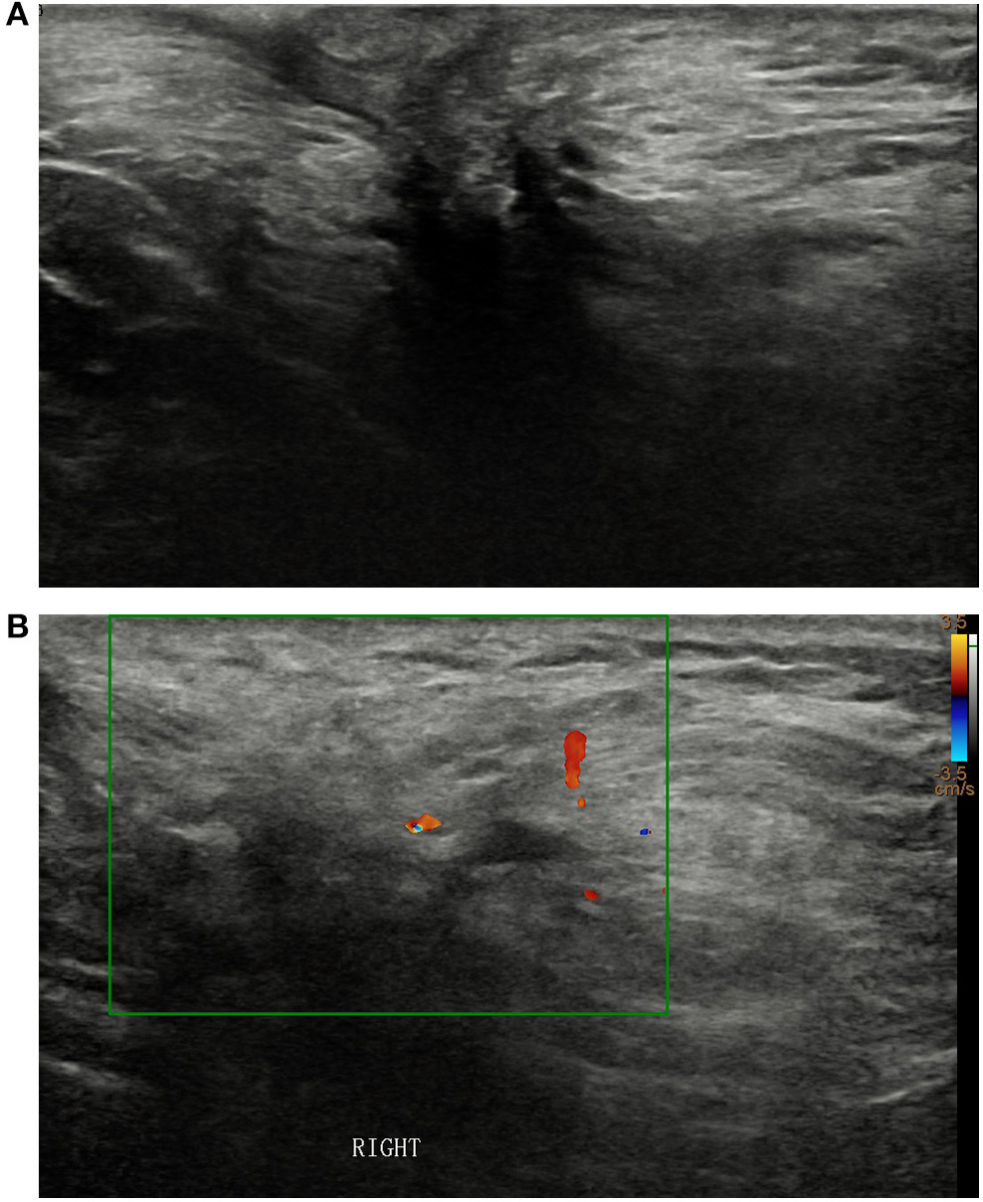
(A) Sonographic examination of the right axilla revealed an irregularly shaped hypoechoic mass with unclear borders and uneven internal echoes. (B) Color Doppler ultrasonography (B) depicted spot-like blood flow signals around the mass.
